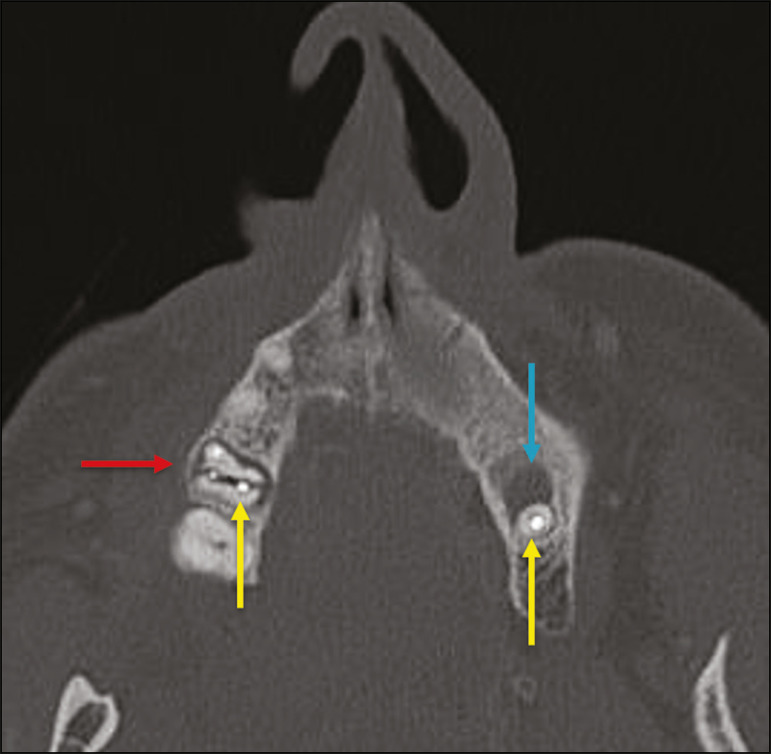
Axial CT of the face showing a longitudinal fracture of the upper right first molar (red arrow) with signs of endodontic treatment (yellow arrows) in some teeth. Note also the periapical disease in the upper left first molar (blue arrow).
